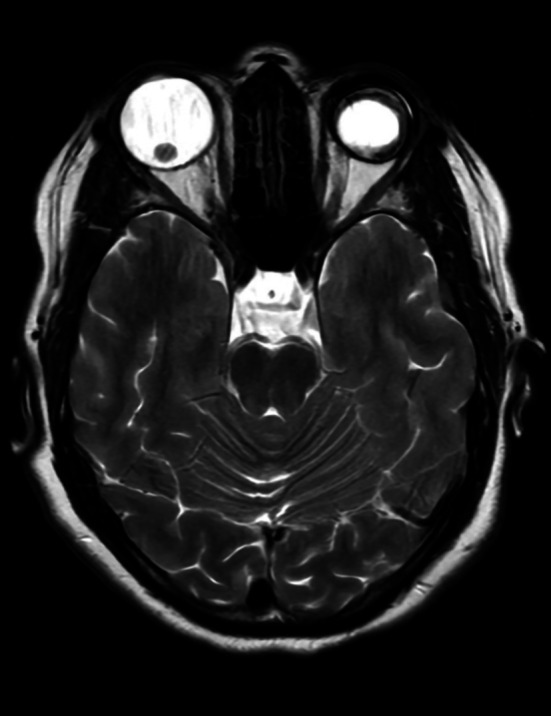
Orbital MRI (T2‐weighted sequence) revealing downward lens dislocation in the right eye and phthisis bulbi in the left eye, characterized by a shrunken, structurally disorganized globe.
